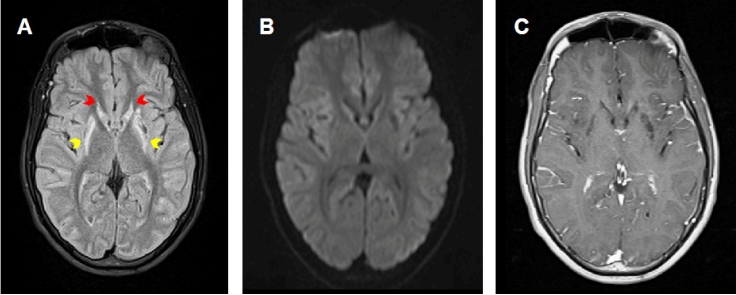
(A) Axial fluid attenuation inversion recovery sequence shows bilateral high signal intensity of the caudate nuclei (red arrows) and putamina (yellow arrows). (B) Diffusion-weighted imaging shows no restricted diffusion. (C) T1-weighted images obtained after contrast injection demonstrate no contrast enhancement.
